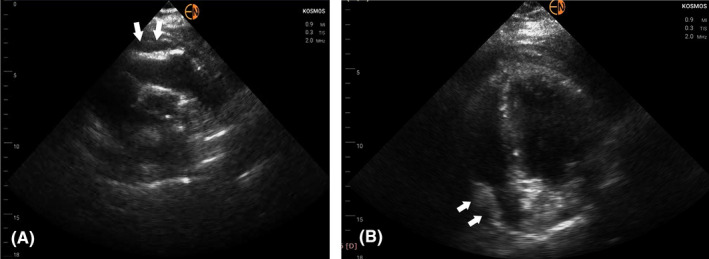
A, Parasternal short‐axis view at the level of aortic valve demonstrating compression of the RV (white arrows); B, Apical four‐chamber view showing compression of the right atrium (white arrows)
